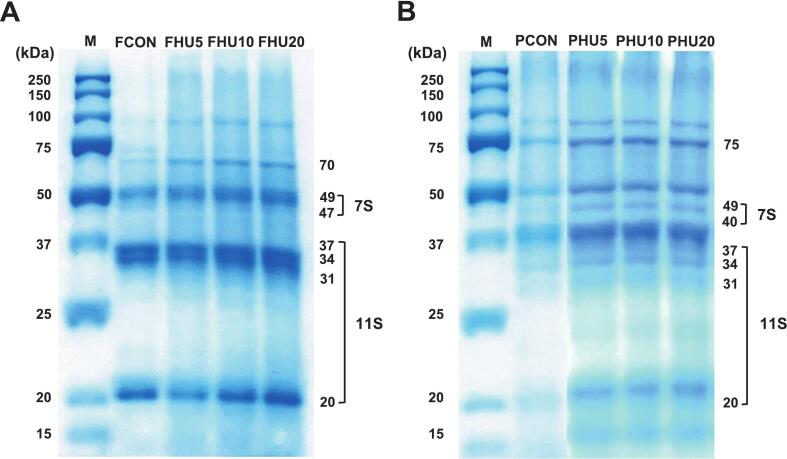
SDS-PAGE of HU-treated legume protein isolates. F, faba bean protein isolates; P, pea protein isolates; CON, untreated 4 % protein dispersions; HU, 4 % protein dispersions sequentially heated and ultrasonicated for 5, 10 and 20 min, respectively.
